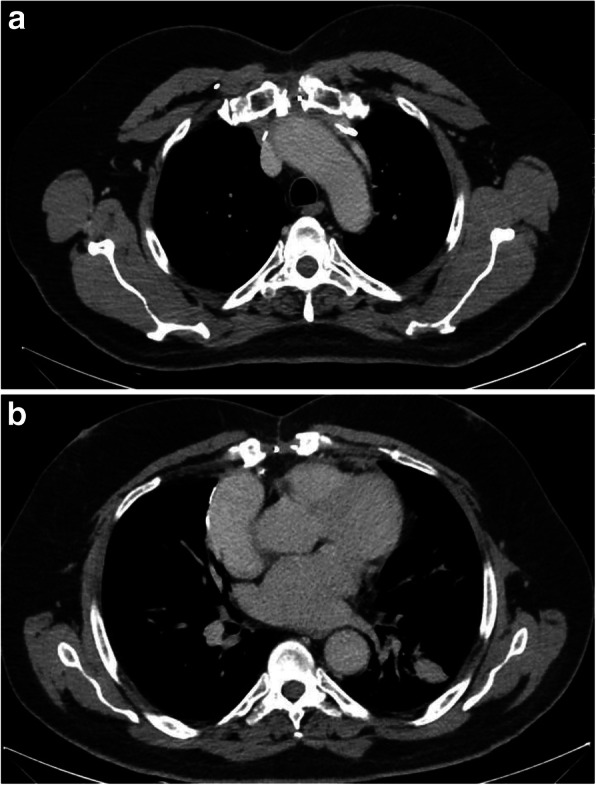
Post-operative chest CT scan, axial view, mediastinal window at the level of the aortic arch (a) and the level of the heart (b) showing complete excision of the anterior mediastinal mass via midline sternotomy
